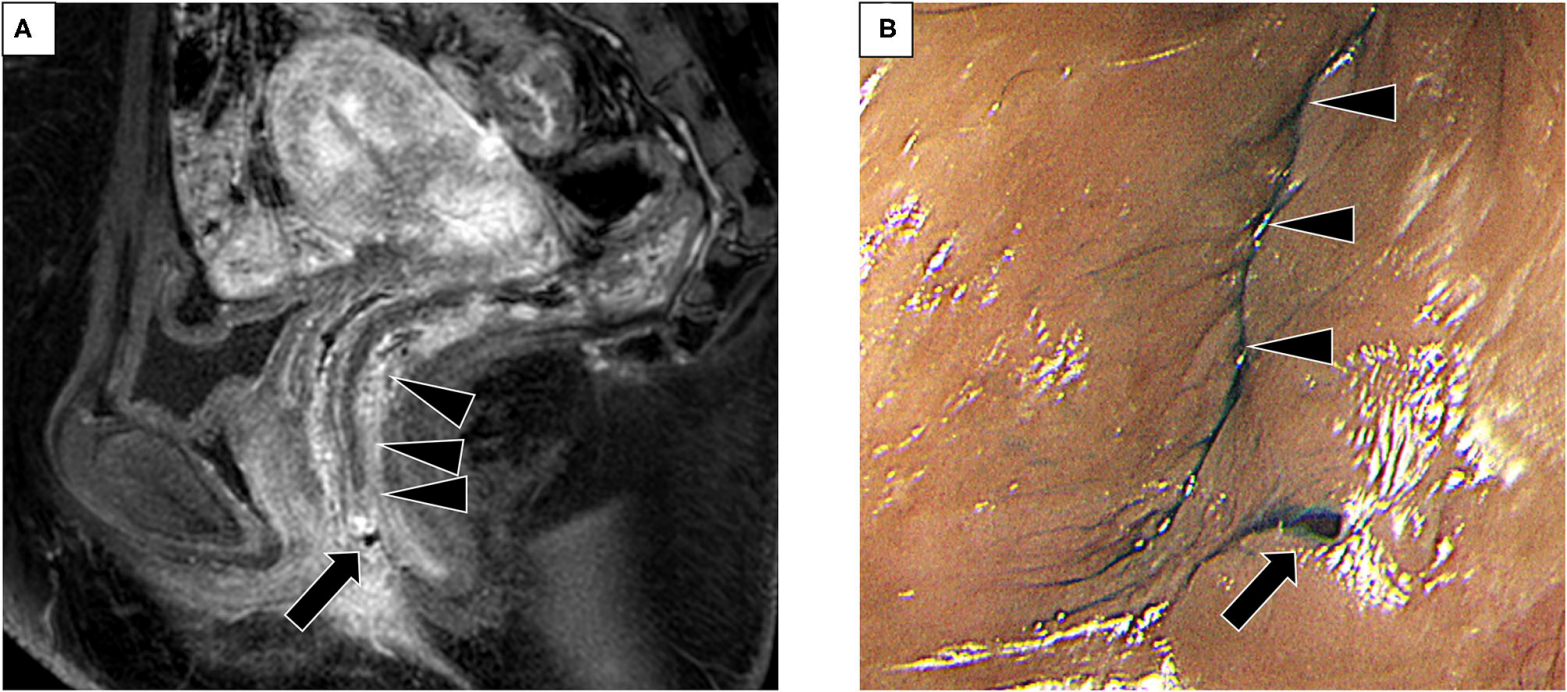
Pre-operative examination for the diagnosis of rectoperineal fistula (A) Magnetic resonance imaging (sagittal view) showing a small tract from the lower rectum. Arrow, small tract. Arrowhead, rectum. (B) Small fistula on the perineal skin visualized after insertion of indigo carmine poured into the rectum (arrow). Arrowhead, vaginal orifice.
